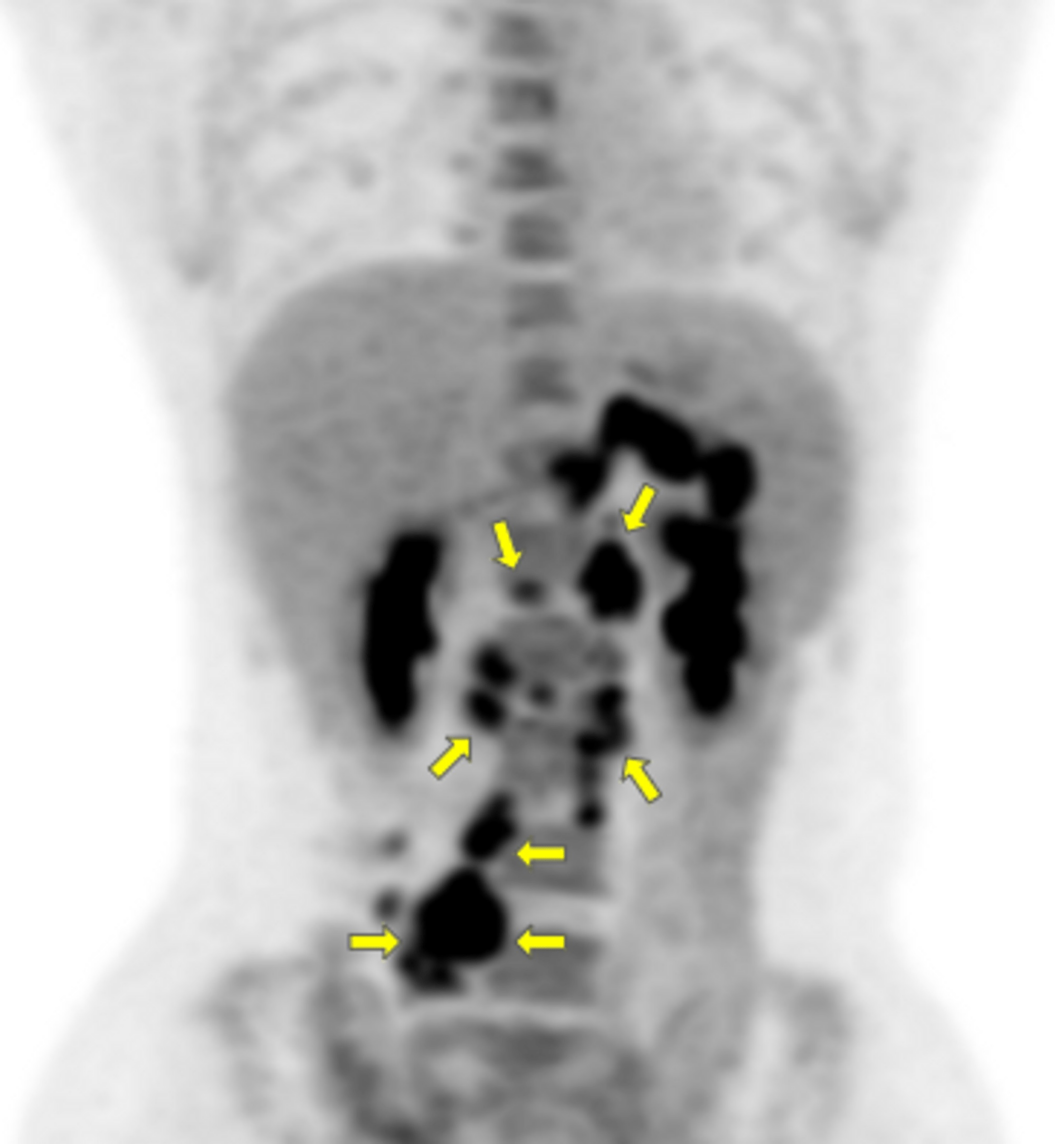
Positron emission tomography (PET) scan with maximum intensity projection (MIP) PET with MIP shows extensive sites of involvement visualized as areas of fluorodeoxyglucose (FDG) uptake in the upper abdomen and mesenteric and retroperitoneal lymph nodes (yellow arrows)
